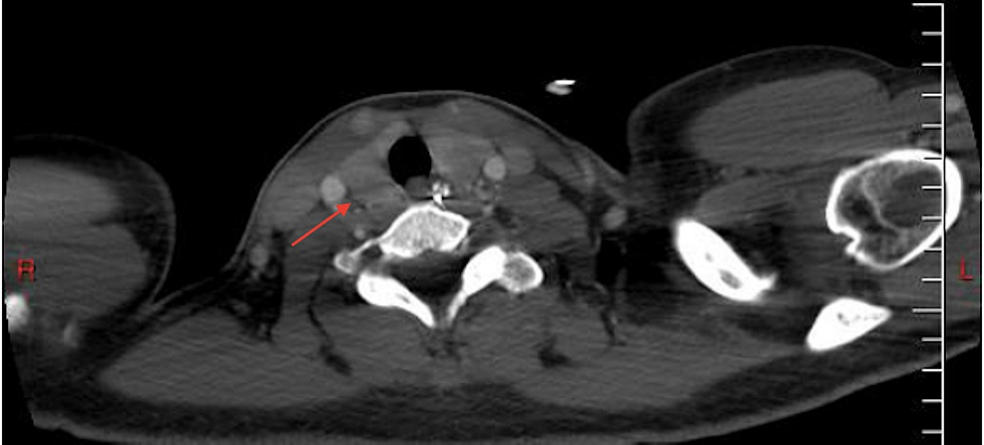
Computed tomography of the abdomen and pelvis, indicating fibroids (red arrow)
